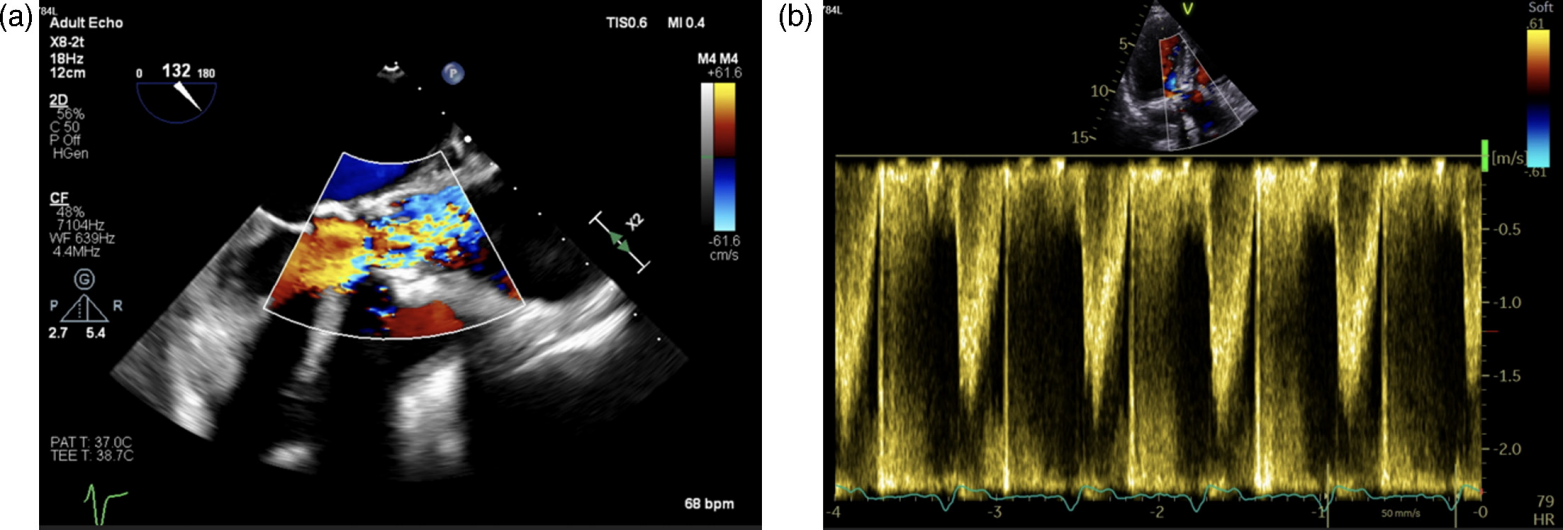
(a) Transoesophageal echocardiography shows moderate transvalvular regurgitation from a suspected tear in the right coronary cusp, with flow reversal in the descending aorta. (b) Continuous-wave Doppler confirming regurgitation with a peak velocity of 3.4 m s^−1^ and a mean gradient of 25 mmHg.
